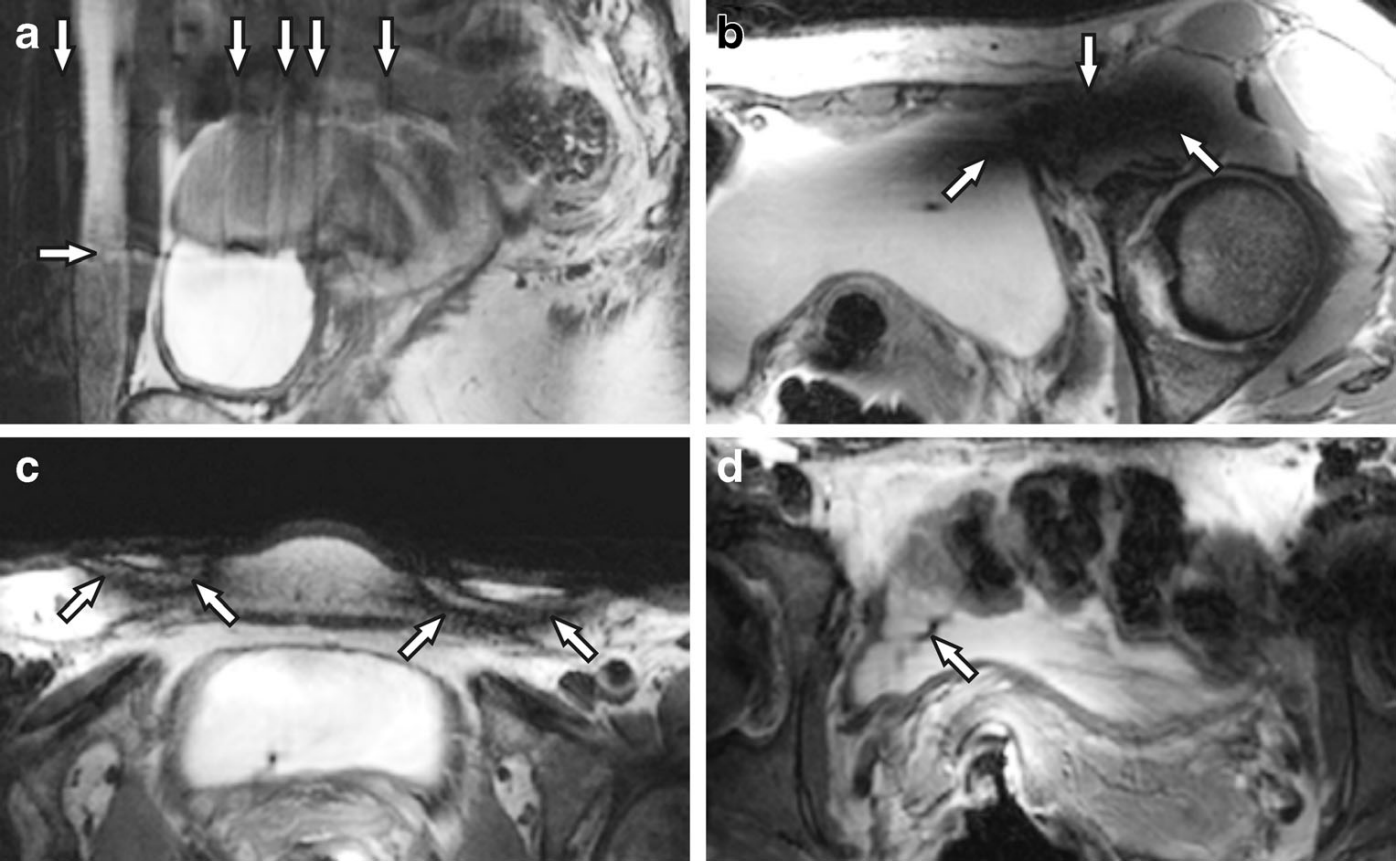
Image artefacts that were encountered on 7.0-T MRI were a motion artefacts, b locally destructive B_1_ interference, c inversion bands due to too much B_1_ under the external transmit/receive antennae and d SENSE reconstruction artefacts. Note the unrelated vaginal tampon (*asterisk*) in c
